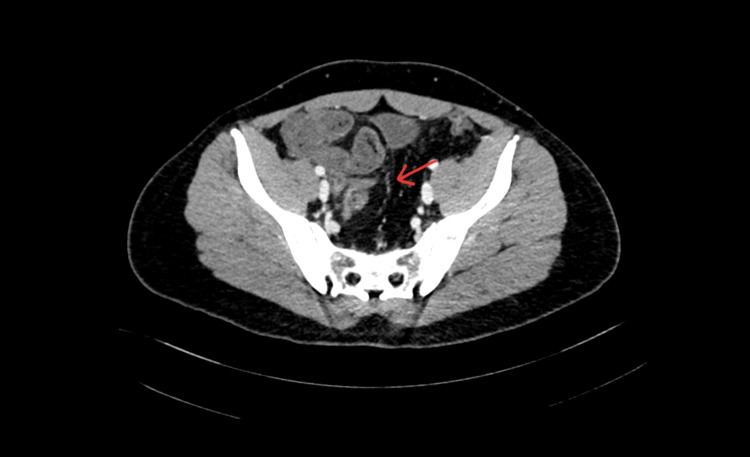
CT of the abdomen and pelvis. A thin membrane surrounding the small bowel is visible (arrow). CT: computed tomography
